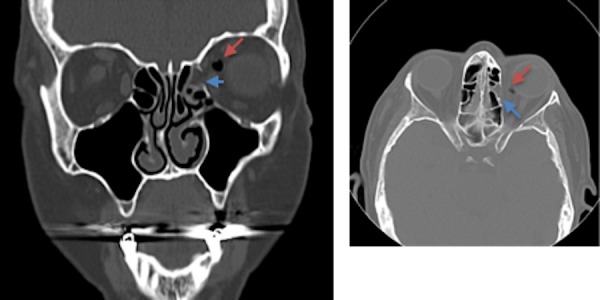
Coronal and axial CT of the facial bones demonstrating a non-displaced left orbital medial wall fracture Coronal (left) and axial (right) CT of the facial bones demonstrates a non-displaced left orbital medial wall fracture (blue arrow) resulting in intra-orbital air. There is minimal sinus opacification of the left medial ethmoid air cell without evidence of an air-fluid level present. An extra-sinus air locule (red arrow) is seen along the supromedial left orbit, likely post-traumatic in nature.
